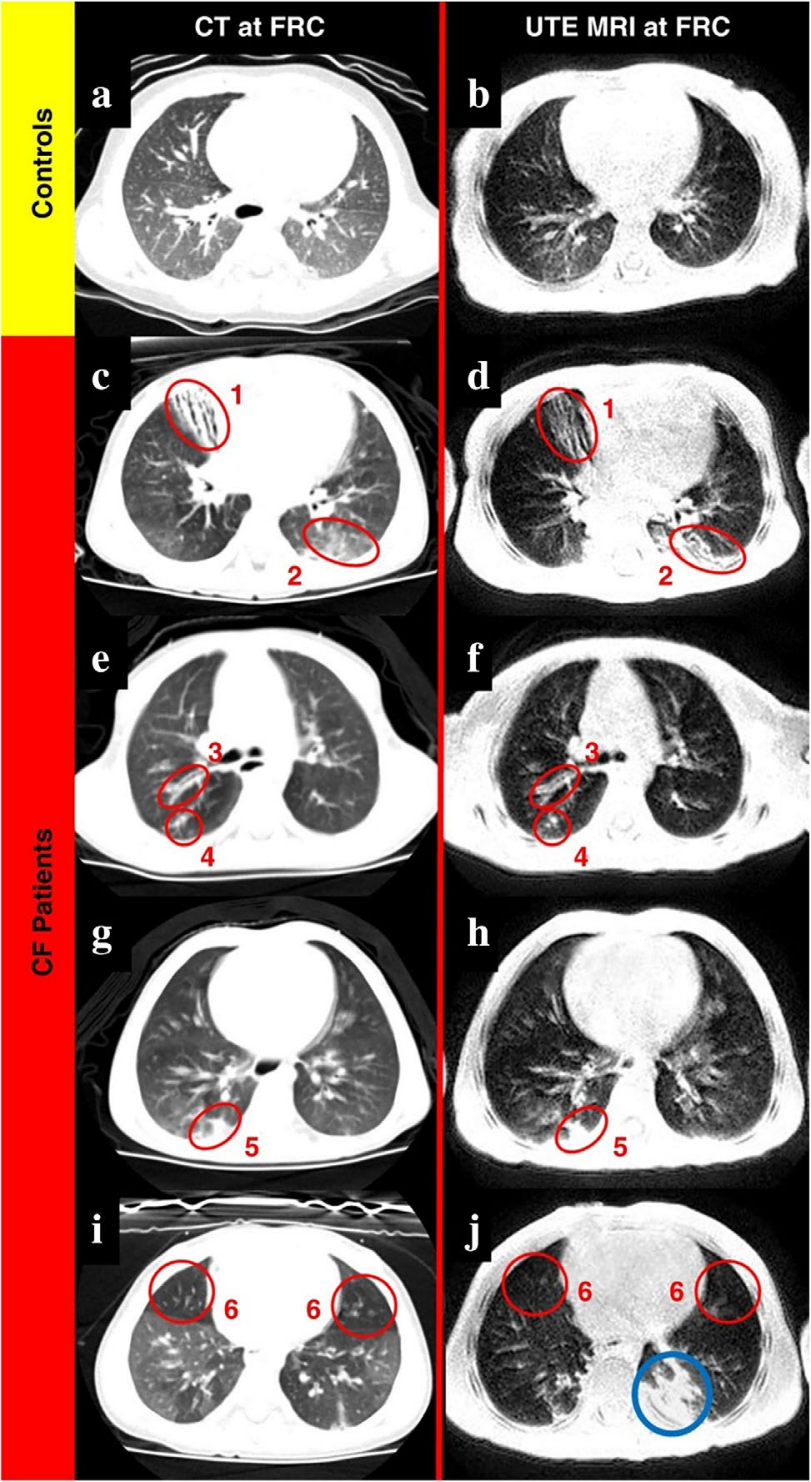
Ultra‐short echo time (UTE) MRI and CT imaging in a healthy control and patients with cystic fibrosis (CF) obtained at functional residual capacity (FRC). Most features apparent on CT can also be observed in UTE MRI. Reprinted with permission of the American Thoracic Society [142]. Copyright © 2016 American Thoracic Society. All rights reserved. Annals of the American Thoracic Society is an official journal of the American Thoracic Society.
